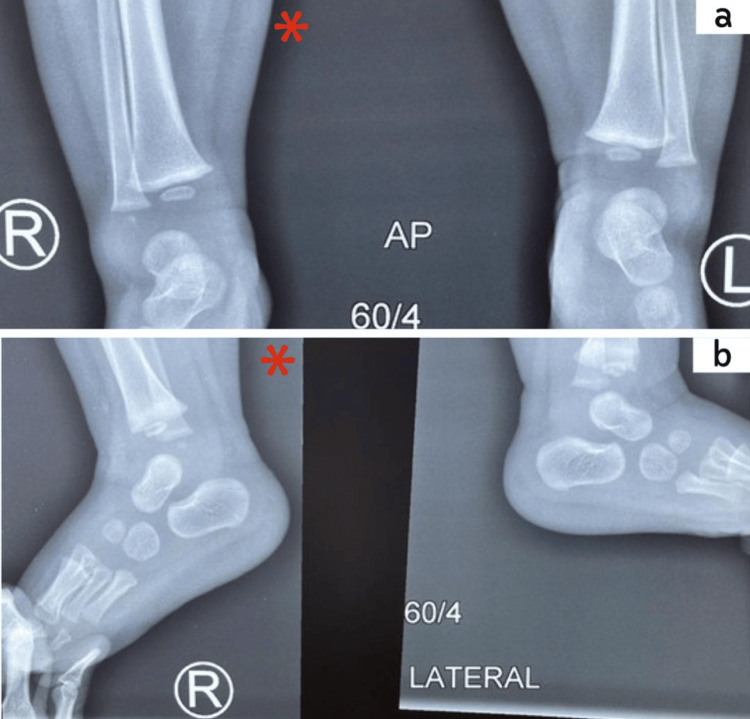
Plain radiographs of both the ankles in anteroposterior and lateral views, labeled a and b, respectively, pathological right ankle, marked with red asterisk, showing no bony abnormality or joint space widening at initial presentation
